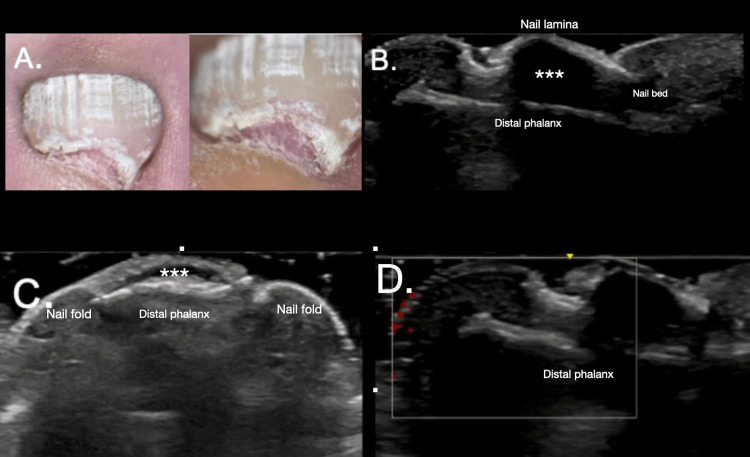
Imaging features of a subungual lesion. (A) Clinical image and LUMIO augmentation show the nail apparatus of the left first toe with a firm, pink lesion located below the nail plate, displacing it upwards into the nail lamina. The patient underwent high-resolution ultrasound evaluation to rule out a glomus tumor. (B-D) High-resolution images of the affected nail’s ungual apparatus reveal a wavy lamina displaced upwards, with a hypoechoic nodule (marked by white asterisks ) occupying the nail bed. The lesion is expansive, extends to the distal phalanx without affecting it, and appears avascular on Doppler evaluation, consistent with a fibrous nail bed. In image C, the nail folds are thickened, indicating chronic inflammation secondary to recurrent onychocryptosis.
